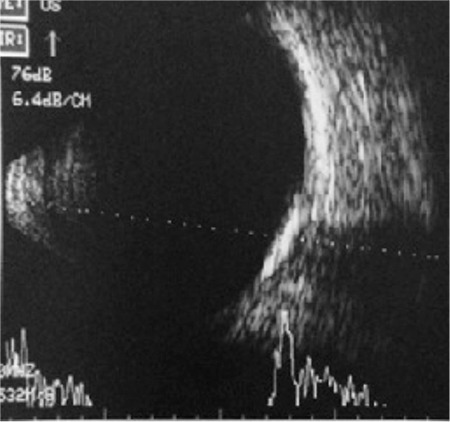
B-scan ultrasonography imaging of the right eye reveals a choroidal lesion causing acoustic shadowing
